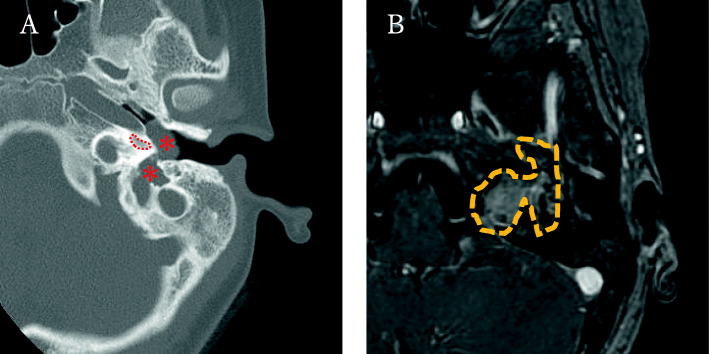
a Preoperative CT and MRI finding. CT shows the tumor was found as a continuous soft shadow from the inner ear to the middle ear by tumor (*). (Dotted area: cochlea). b MRI shows the tumor (dotted area) extends from the inner ear to the middle ear as well as the internal auditory canal, presenting as low signal on contrasted T1
